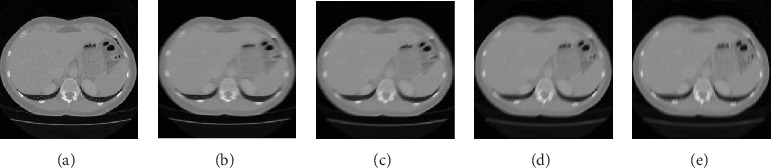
The results of different blurred angle of fatty liver patient. (a) Original CT image. (b) Blurred angle of 0°. (c) Blurred angle of 30°. (d) Blurred angle of 60°. (e) Blurred angle of 90°.
